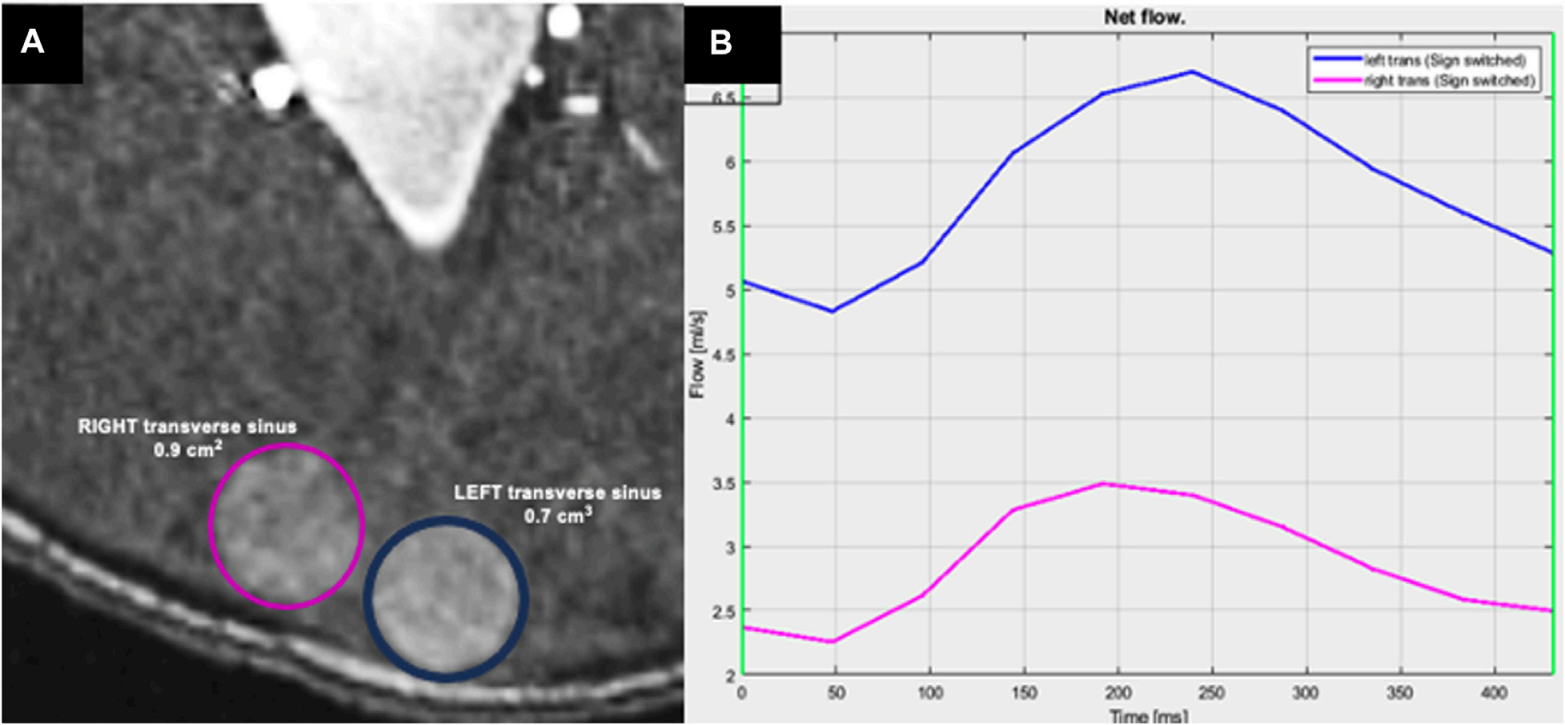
Measurement of flow rate within the transverse sinuses by 2D PCMRI. (A) Axial TOF MRI showing the location of measurements with color corresponding to (B) the plot showing the flow rate at the left (blue) and right (pink) transverse sinuses (division downstream to the confluence of sinuses) as measured by 2DPCMRI. Plot in (B) shows the flow rate over a sampled cardiac cycle, with a higher flow rate through the left transverse sinus at this segment.
